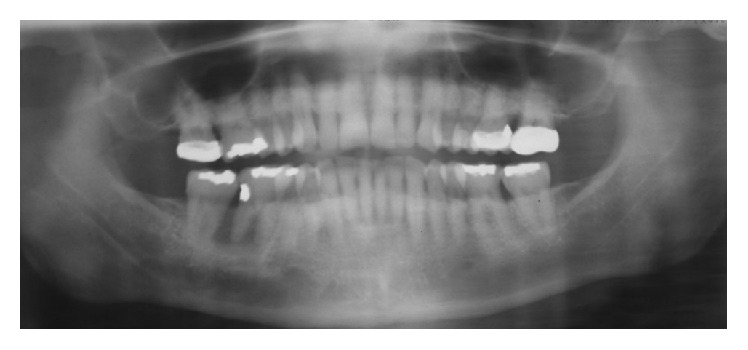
Panoramic radiograph at the initial appointment (#30 was extracted by the referring dentist).
